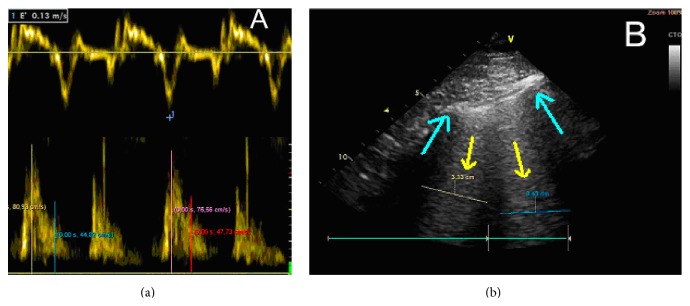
(a) Top: Doppler tissue of the lateral mitral annulus showing the value of *e*′. Bottom: Doppler mitral filling. Together both images show a pattern of normal mitral filling estimate normal filling pressure (value *E*/*e*′ < 8). (b) The picture shows lung ultrasound realized with a sectorial probe in the patient's right chest, between fourth and fifth ribs in midclavicular line. Figure shows presence of vertical B lines > 3 mm (yellow arrows), indicative of pulmonary edema. Blue arrows show rib shadows.
